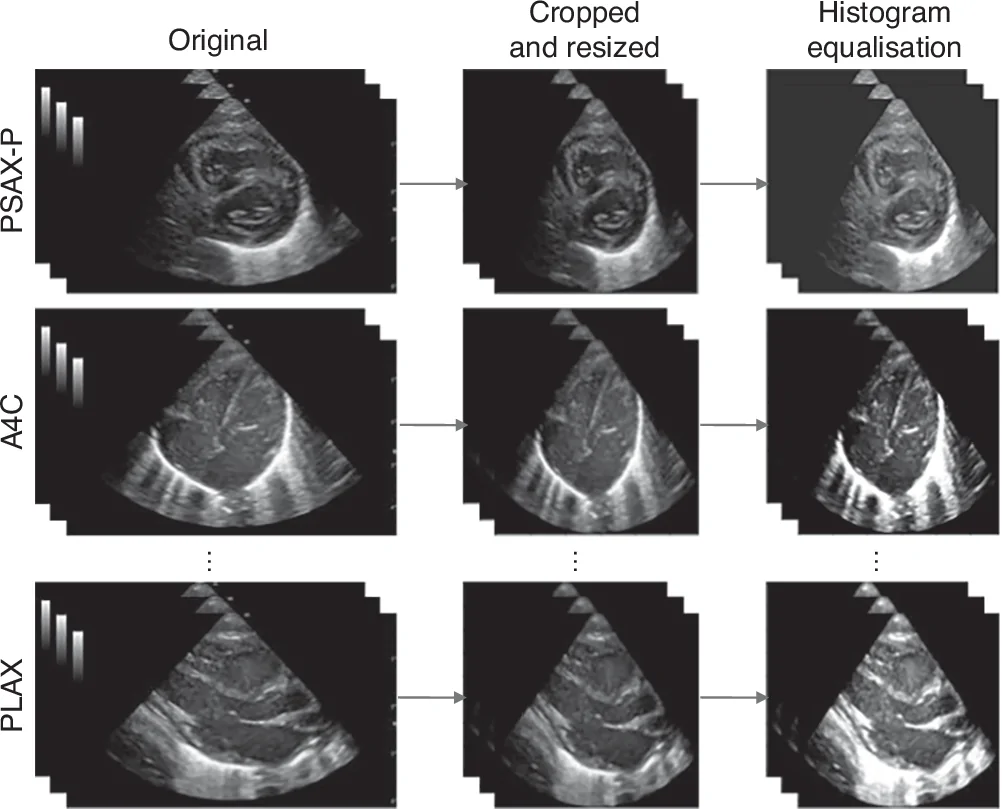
Dataprocessing of the echocardiographic videos including cropping and resizing the videos in the first step followed by histogram equalization. PSAX-P parasternal short axis view, A4C apical four chamber view, PLAX parasternal long axis view.
